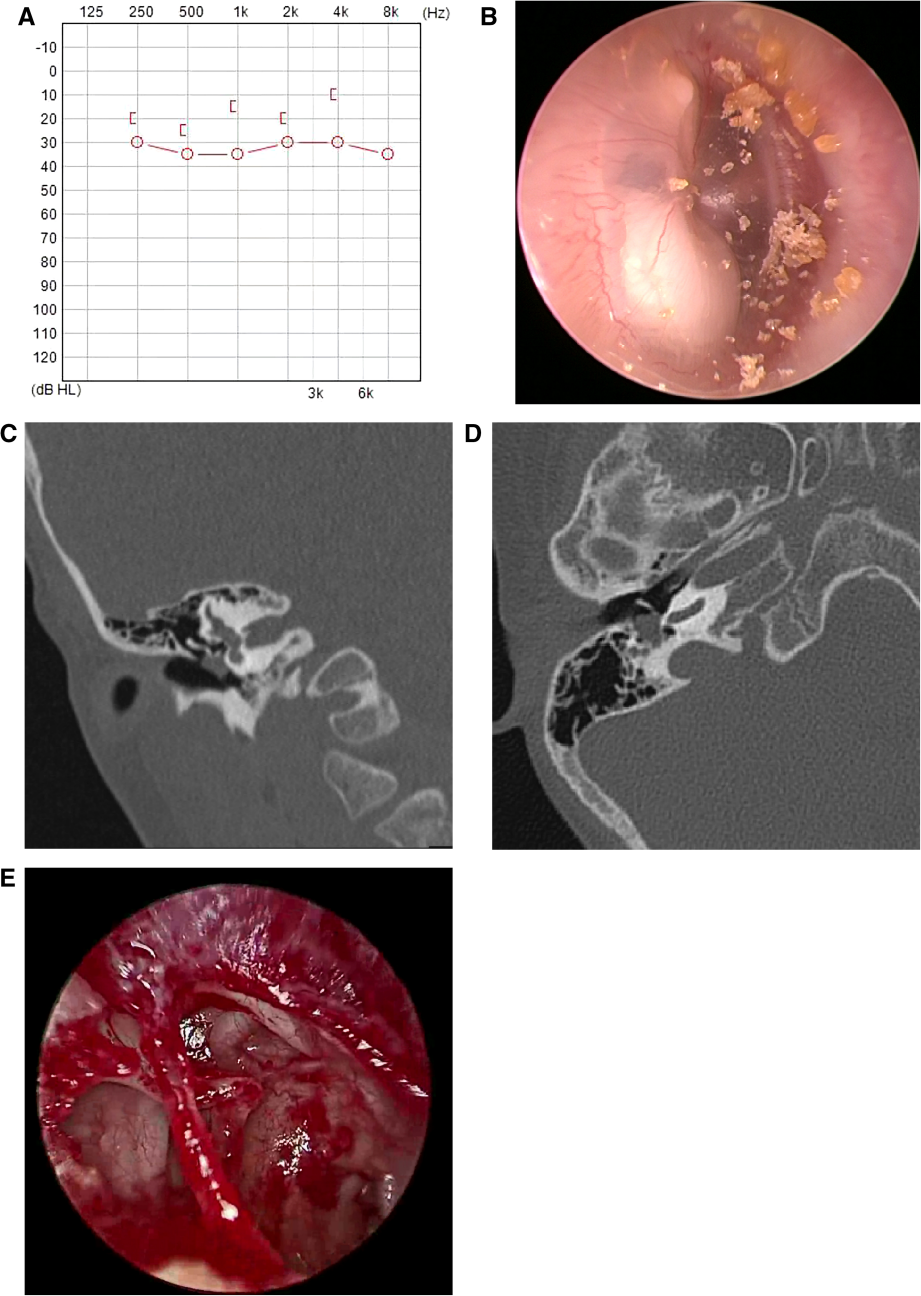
Typical case of stage II CMEC. (A) An audiogram revealed mildly abnormal hearing before surgery; (B) Endoscopic findings revealed a white mass involving the post superior and inferior quadrants of the tympanic membrane; (C,D) CT showing soft tissue density in the right side of the middle ear, without involving the mastoid; (E) After the complete removal of the congenital cholesteatoma, the ossicular chain was intact.
